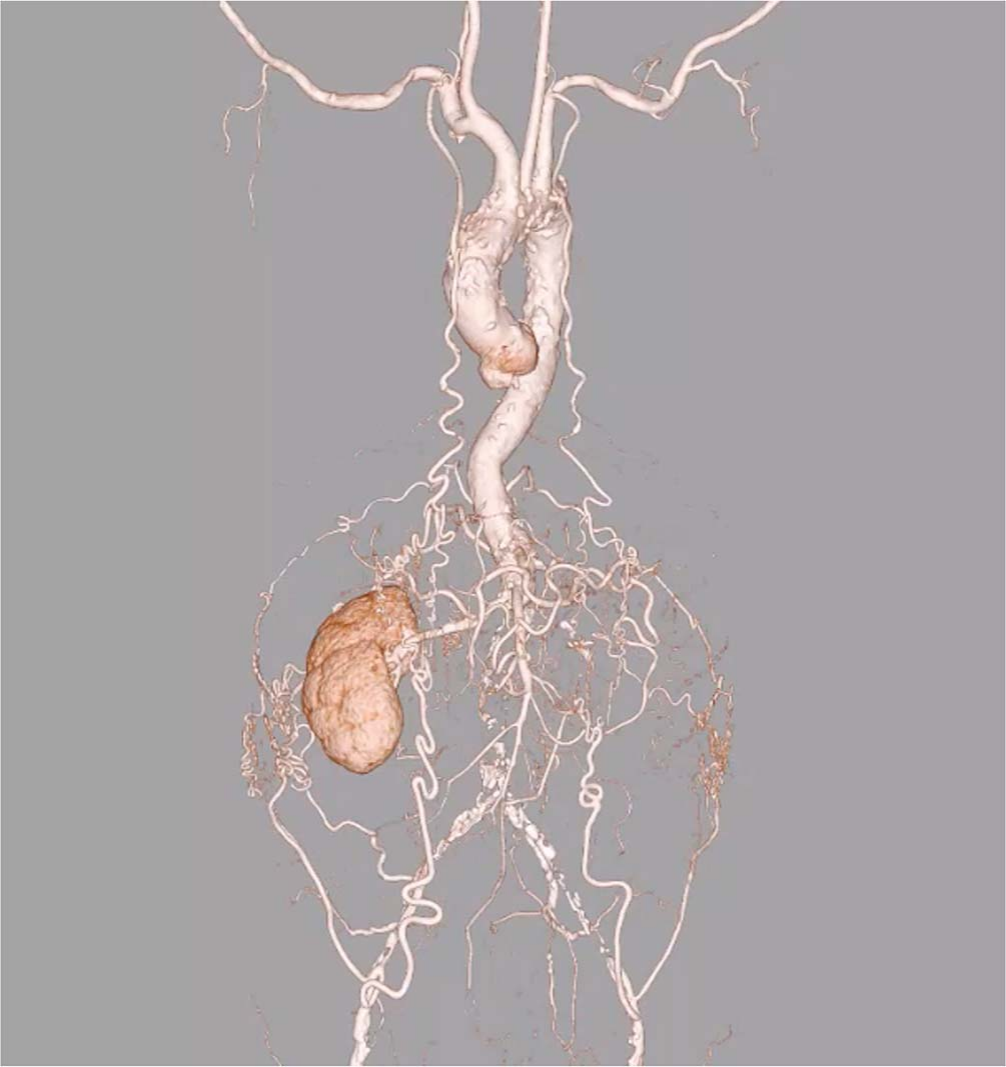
Preoperative 3D CT angiography of the entire vasculature. Preoperative 3D CT angiography image of the entire vasculature demonstrates that the ITAs are an important source of blood flow to the lower limbs.
